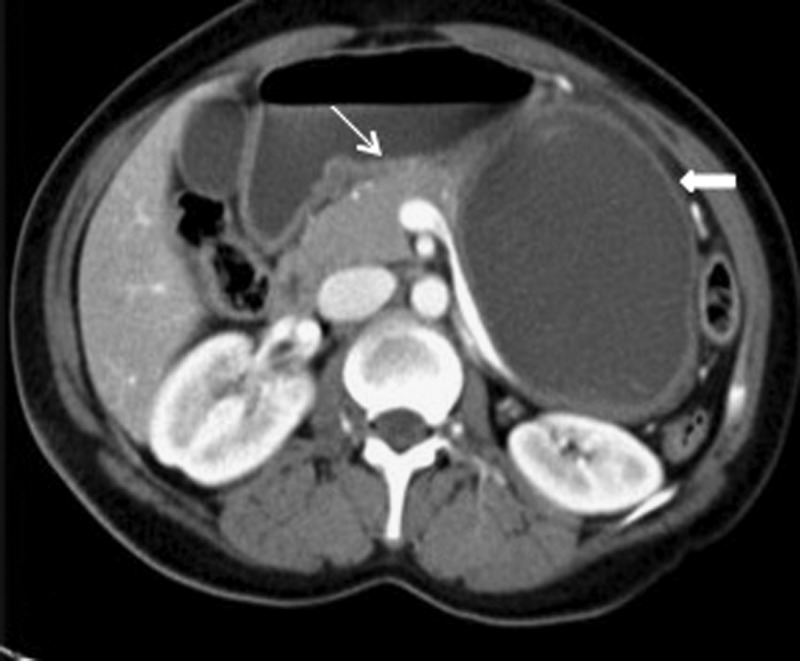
Multidetector computed tomography axial image showing a well-defined unilocular nonenhancing cystic structure (bold white arrow) arising from the distal body and the tail of the pancreas, with internal hyperdense foci. The normal enhancing pancreatic parenchyma is seen proximally (thin white arrow).
